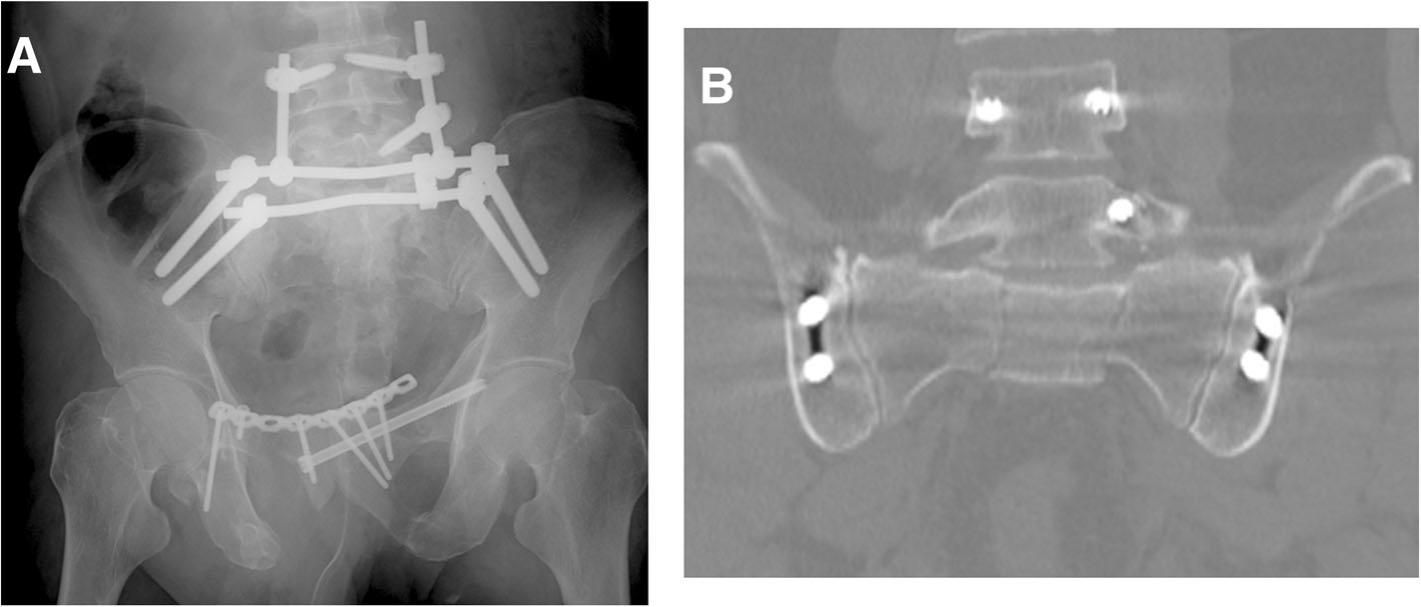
Radiograph and computed tomography (CT) images after surgery. a Anteroposterior radiograph, showing the left L5 pedicle screw, after secondary surgery, which is used to apply distraction to the fracture site (in combination with traction of the left lower limb). b Coronal CT after secondary surgery, showing the residual 5–7.5-mm residual vertical dislocation (cranial direction), but with resolution of the left L5 nerve root pain
